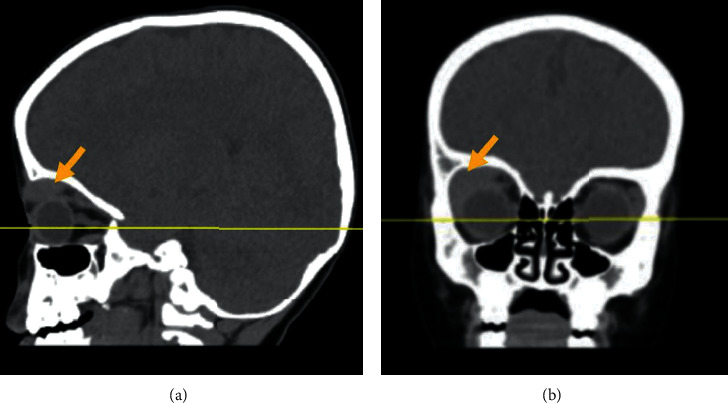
(a, b) A noncontrast computed tomographic scan of the orbits for the reported patient. A noncontrast computed tomographic scan of the orbits demonstrated a well-defined right intraorbital, extraconal, slightly hyperdense lesion noted at the superior and lateral aspect of orbit, abutting the lacrimal gland. It is indenting the superior rectus muscle and eye globe. No defined bone erosion could be seen.
